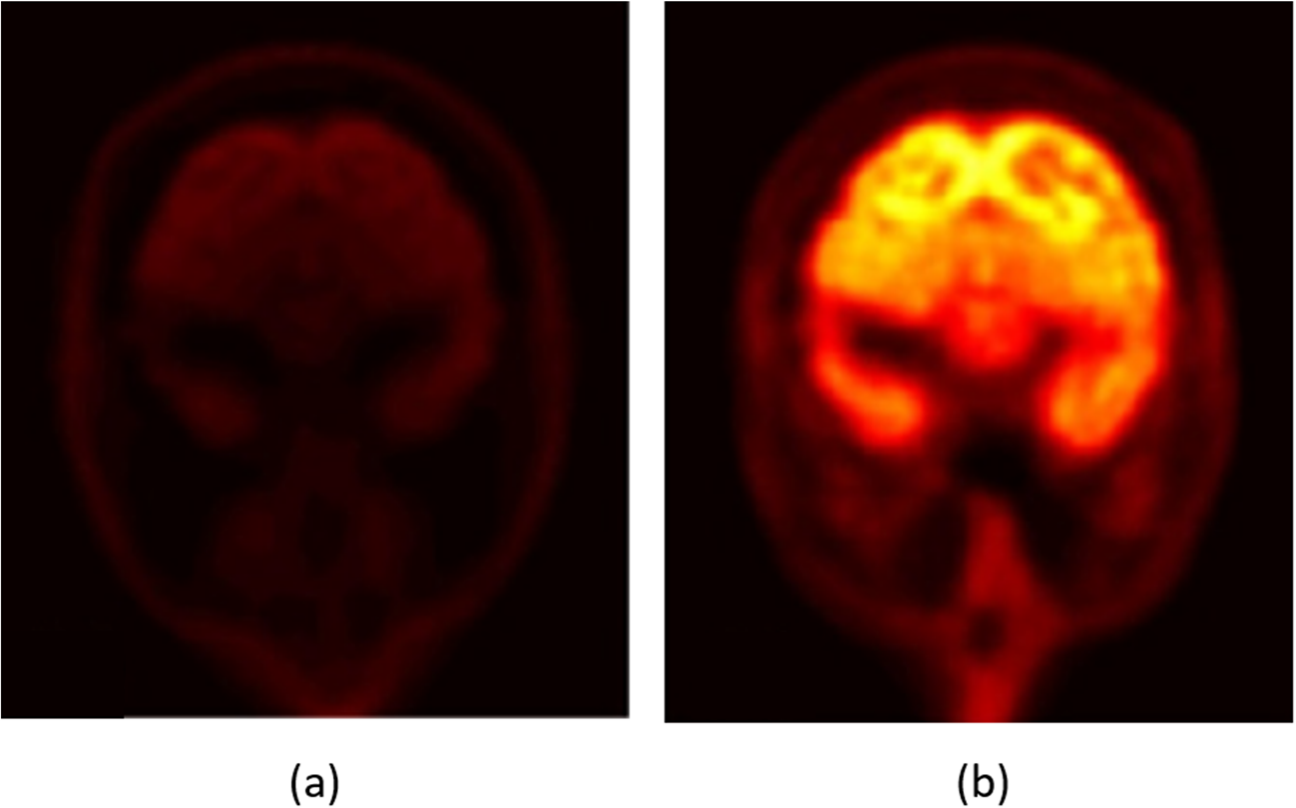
A reconstructed PET image without attenuation correction (a), and with attenuation correction (b) using the [(18)F]-fluorodeoxyglucose ((18)F-FDG) radiotracer. Adopted form [4]
